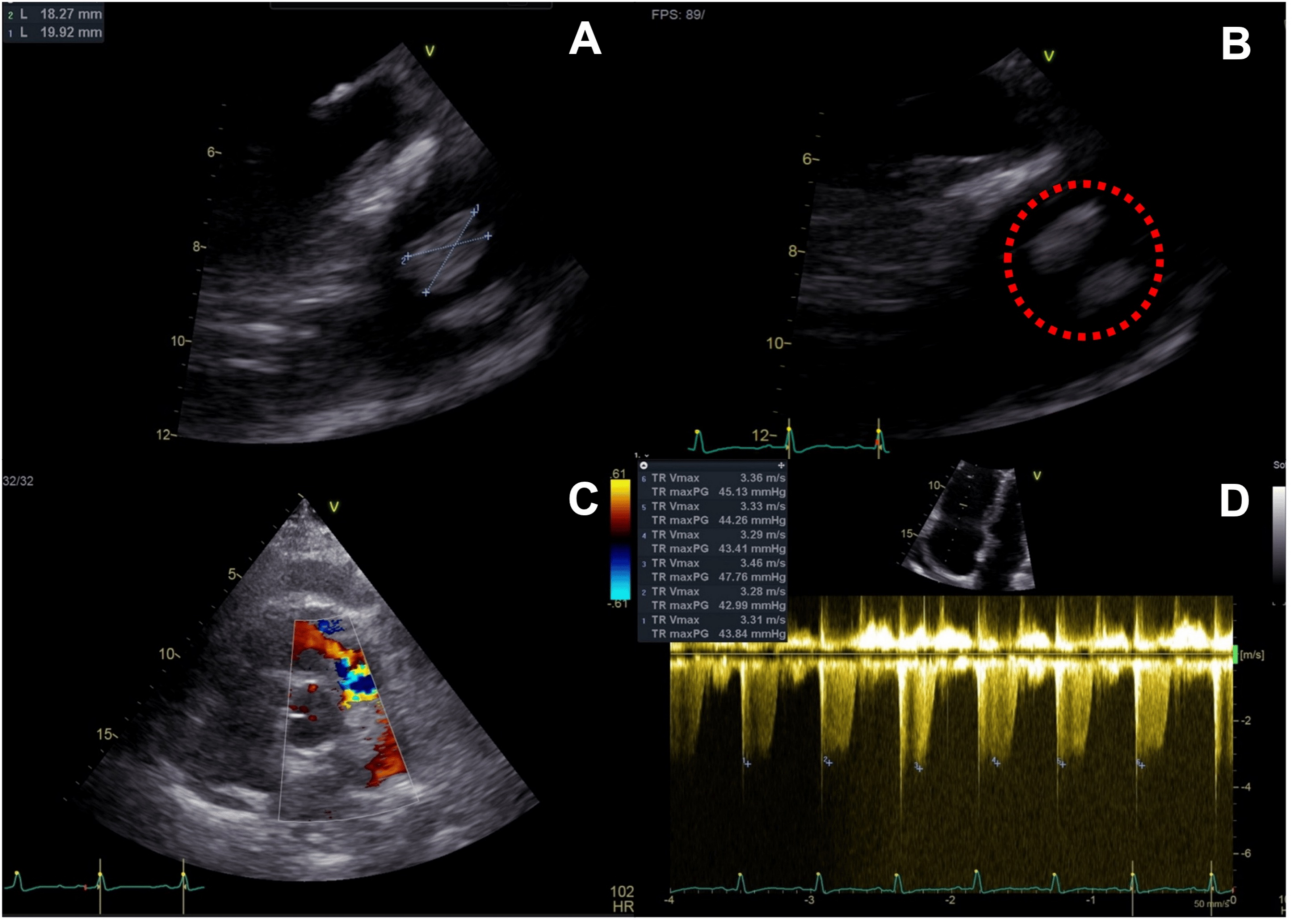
Pulmonary valve vegetation on echocardiography. (A and B) Transesophageal echocardiography revealing pulmonary valve vegetation (red circle) with approximately 20 mm diameter. (C) Transthoracic echocardiography documenting significant pulmonary valve regurgitation with color Doppler. (D) Right ventricle dilation with peak systolic transtricuspid pressure gradient of 45 mmHg.
